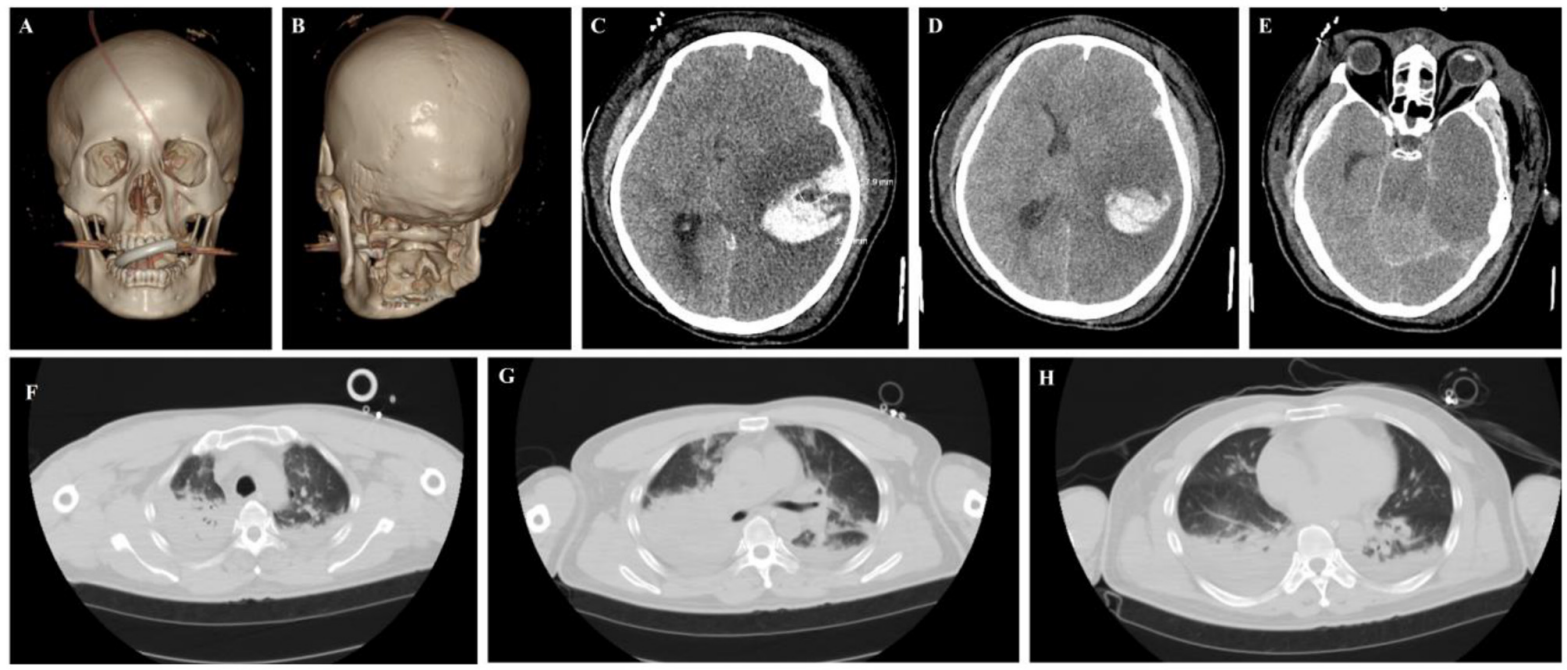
Critical Patient 9: cranial and thoracic imaging examinations. (A–E) Head CT reveals left temporal lobe hemorrhage with edema and compression of the lateral ventricle. (F–H) The chest CT scan showed bilateral lung exudation and consolidation, along with contusion.
